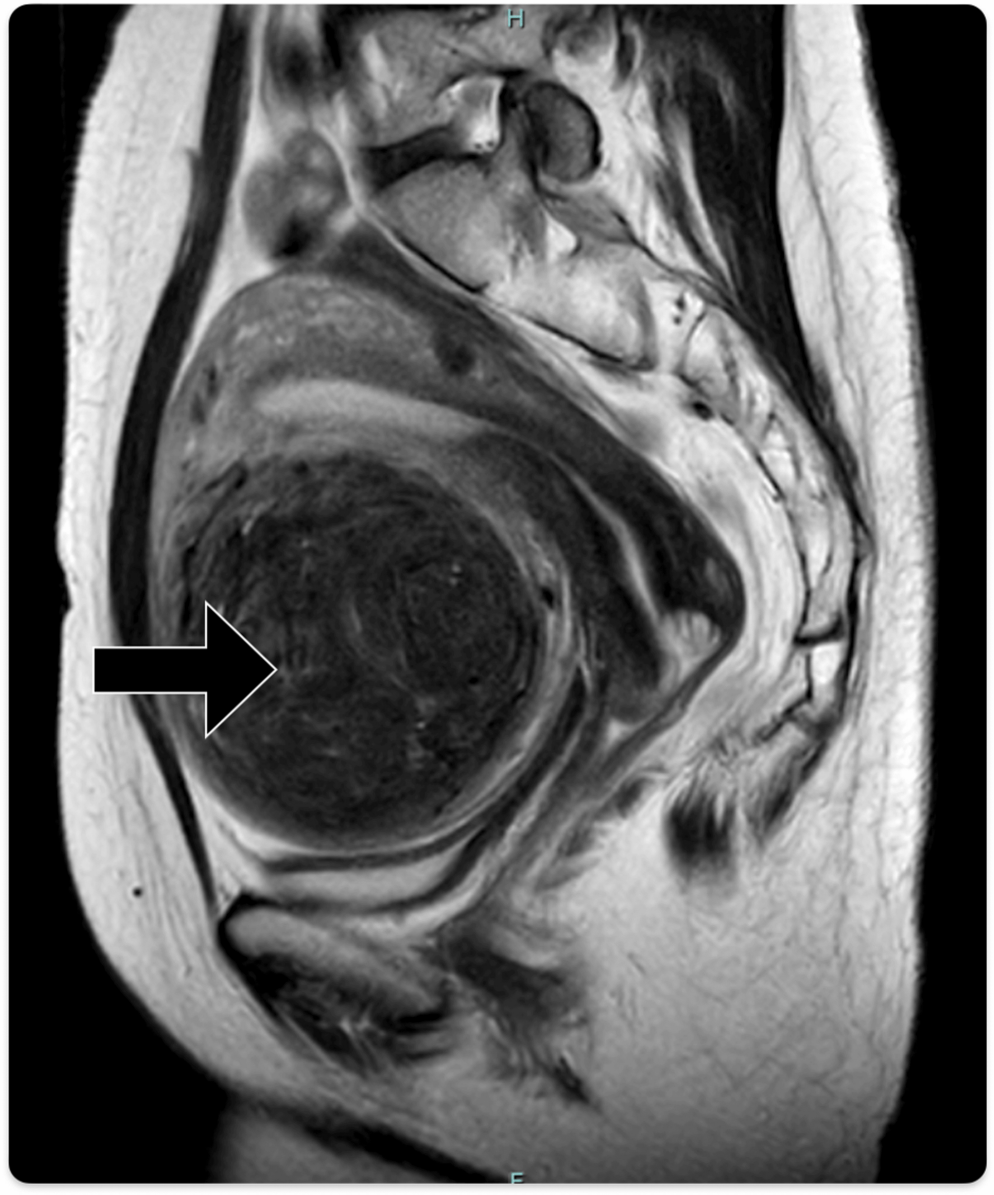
Pre-TLH T2-weighted MRI (sagittal view) demonstrating a low-intensity 12 cm mass (arrow) located on the anterior wall of the uterus. TLH: total laparoscopic hysterectomy
